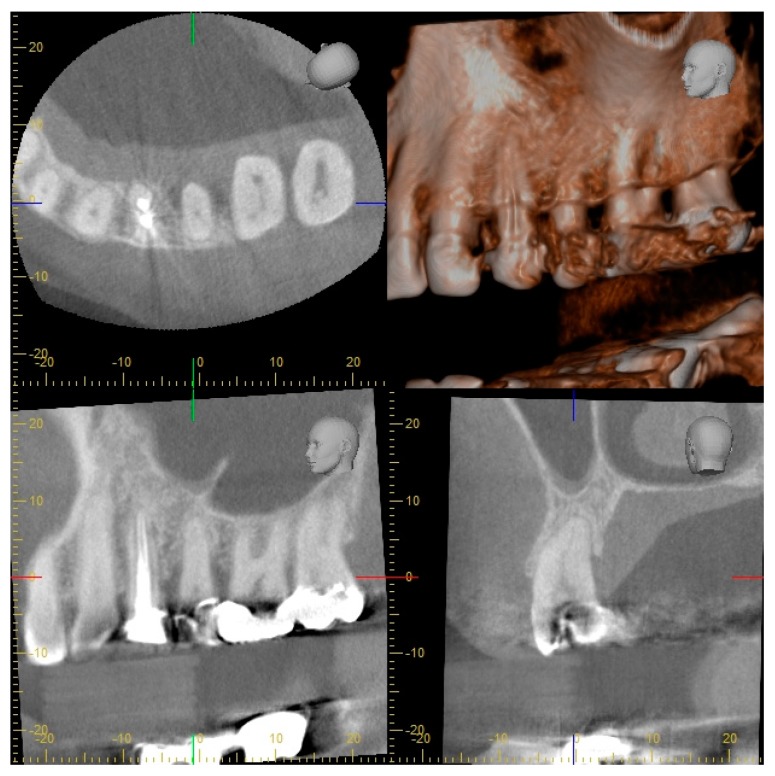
Part of full-mouth cone beam computed tomography (CBCT) with horizontal, sagittal and transversal sections of the first and second left maxillary premolars and molars.
